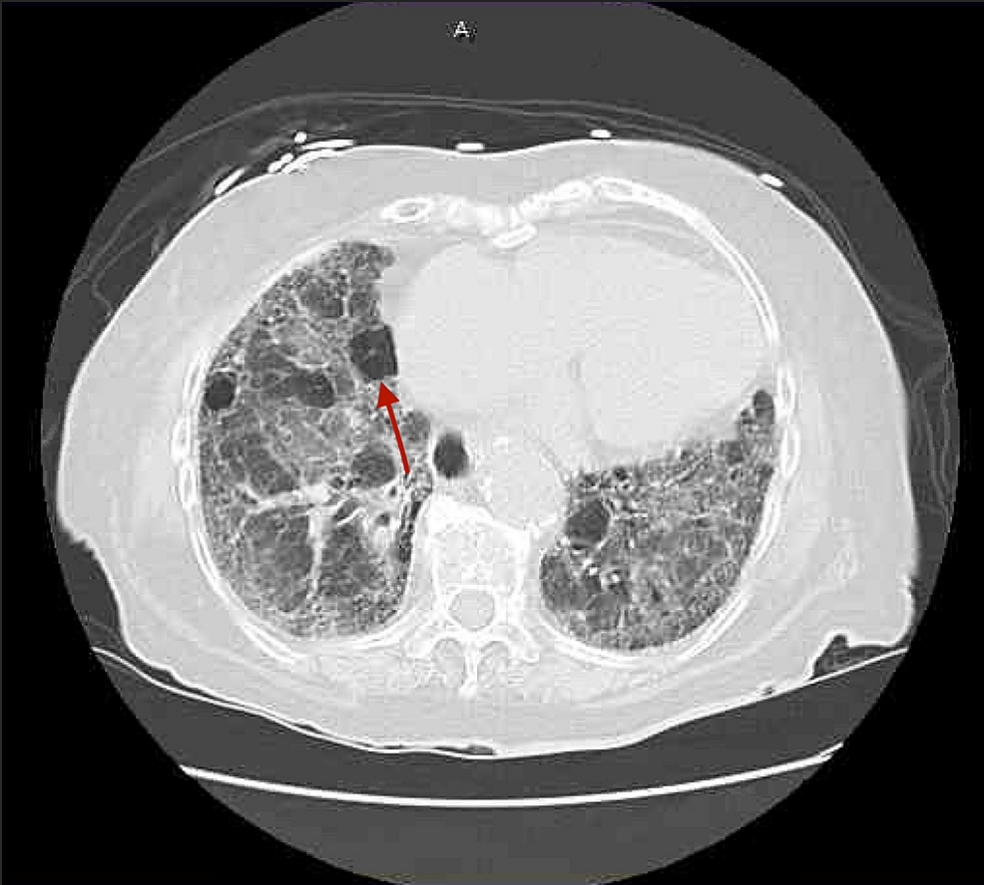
Chest CT without contrast showing air spaces in the lungs (red arrow).
